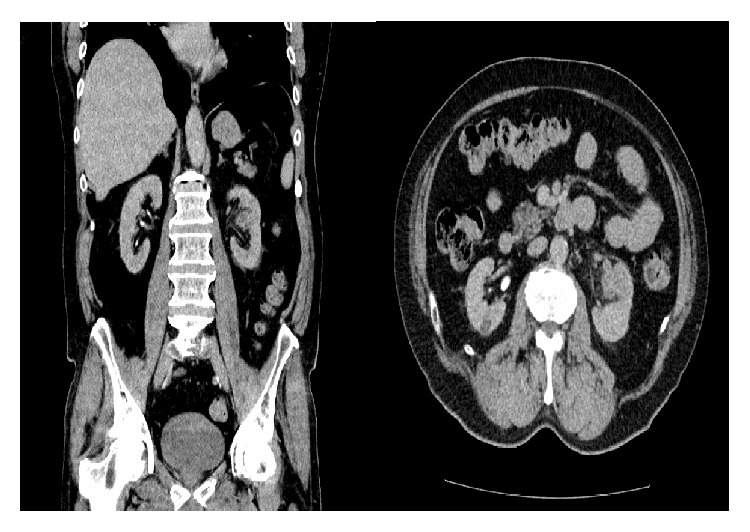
CT imaging of the mid pole solid mass of the left kidney.
